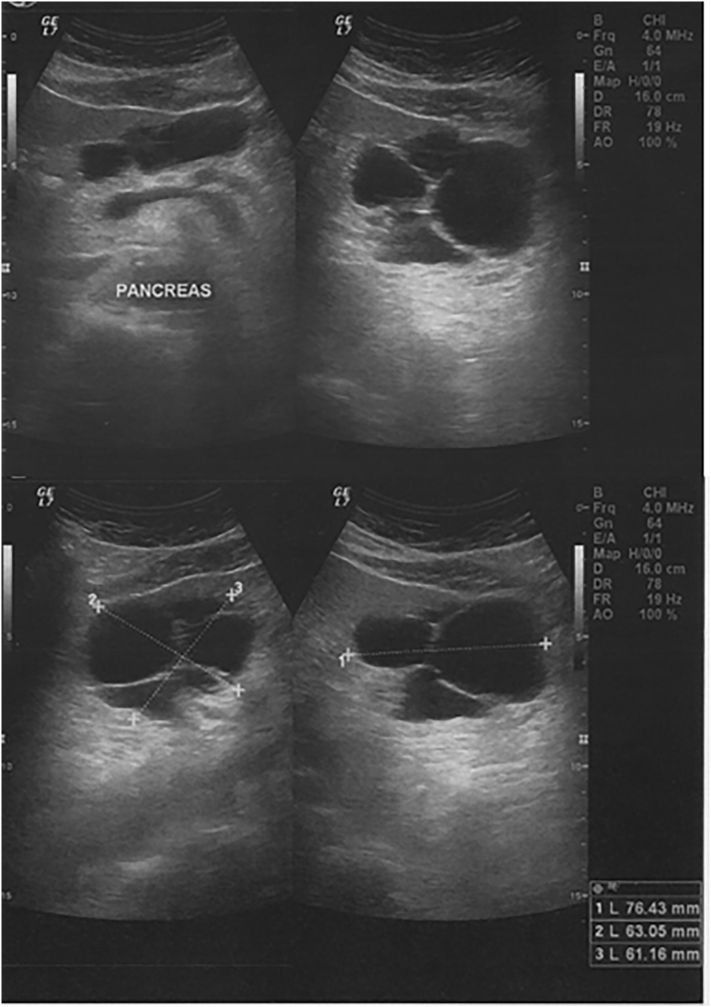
Ultrasound showing a polycystic lesion with fine septa, measuring approximately 76 × 63 × 61 mm, without calcifications or vegetations, located between the pancreas and the left liver lobe.
